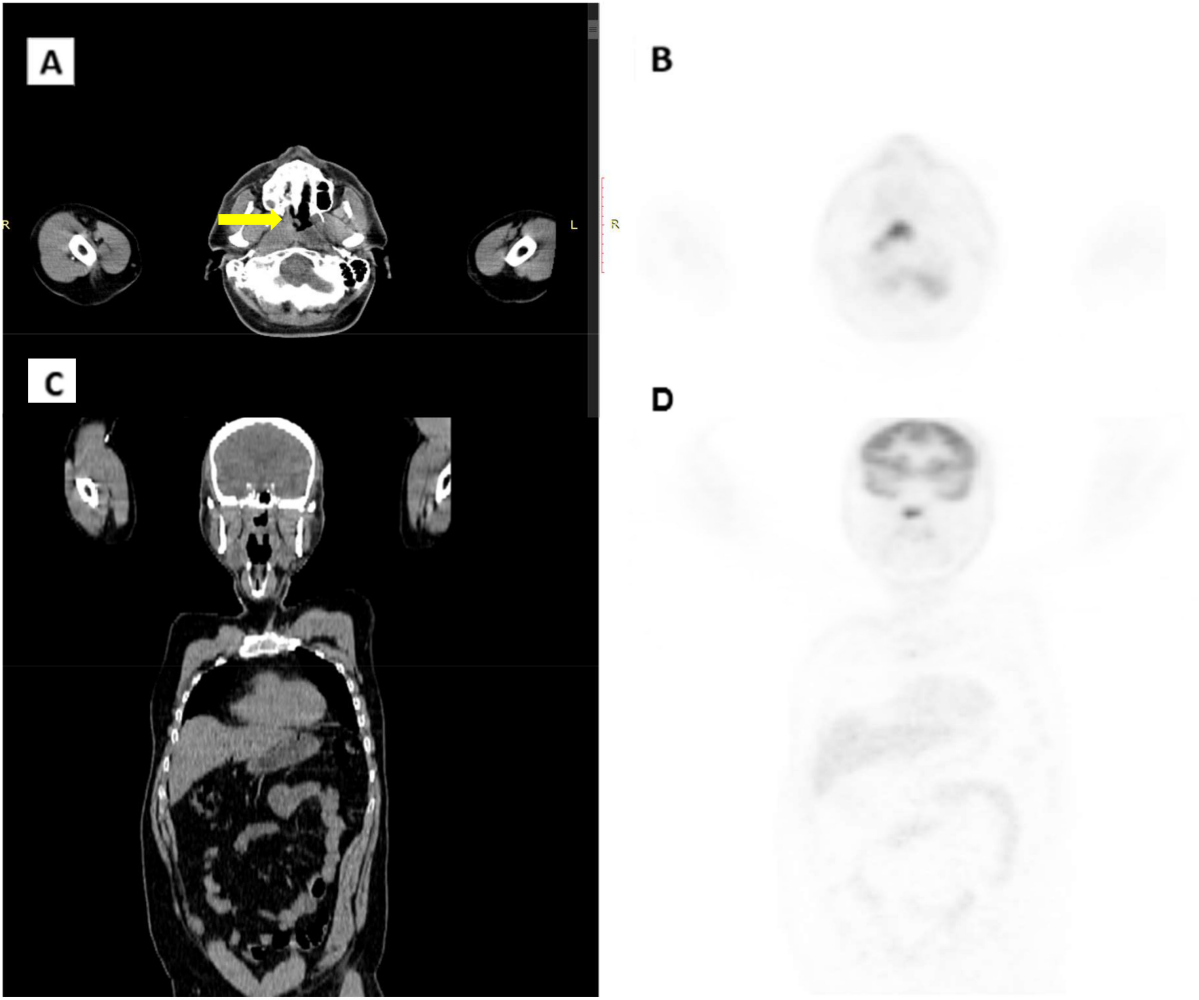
CT/PET scan in June 2022. (A) CT axial scan. (B) CT coronal scan. (C) PET axial scan. (D) PET coronal scan. Arrow indicates pathological thickening of the nasopharynx.
